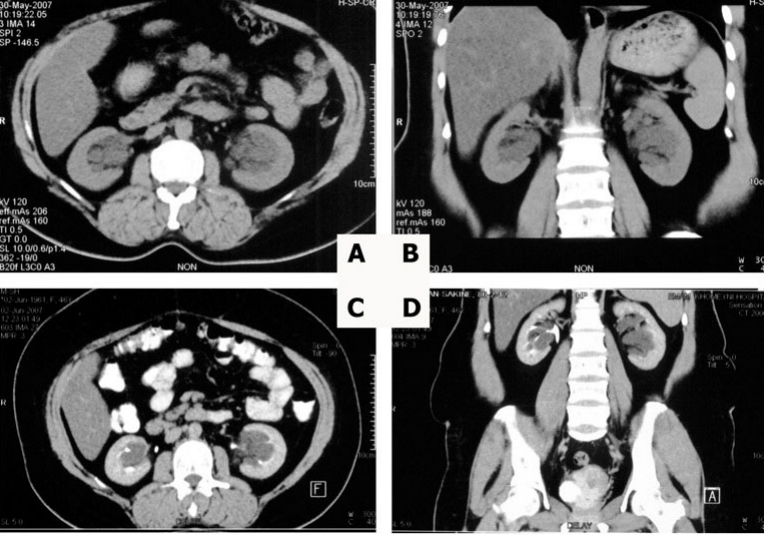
Dilated pelvises seems to be due to hydronephrosis in a non-enhanced CT scan in axial (A) and sagittal reconstruction (B); only one separate parapelvic cyst is detected with this modality. Enhancement of parapelvic cysts septa and infundibuli after contrast injection in axial (C) and sagittal reconstruction (D).
